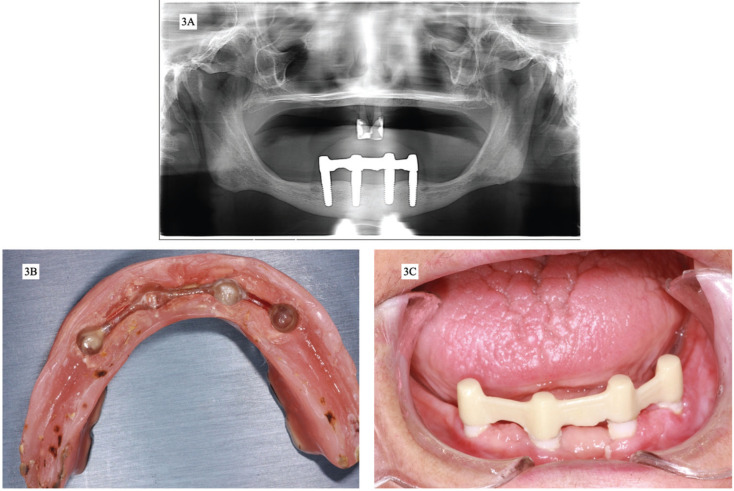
A. Orthopantomography after 12 years of implants placement, B. Overdenture after 12 years of use and before plastic matrices replacement and prophylaxis, C. Zirconia implants and bar after 12 years of placement and before routine prophylaxis.
